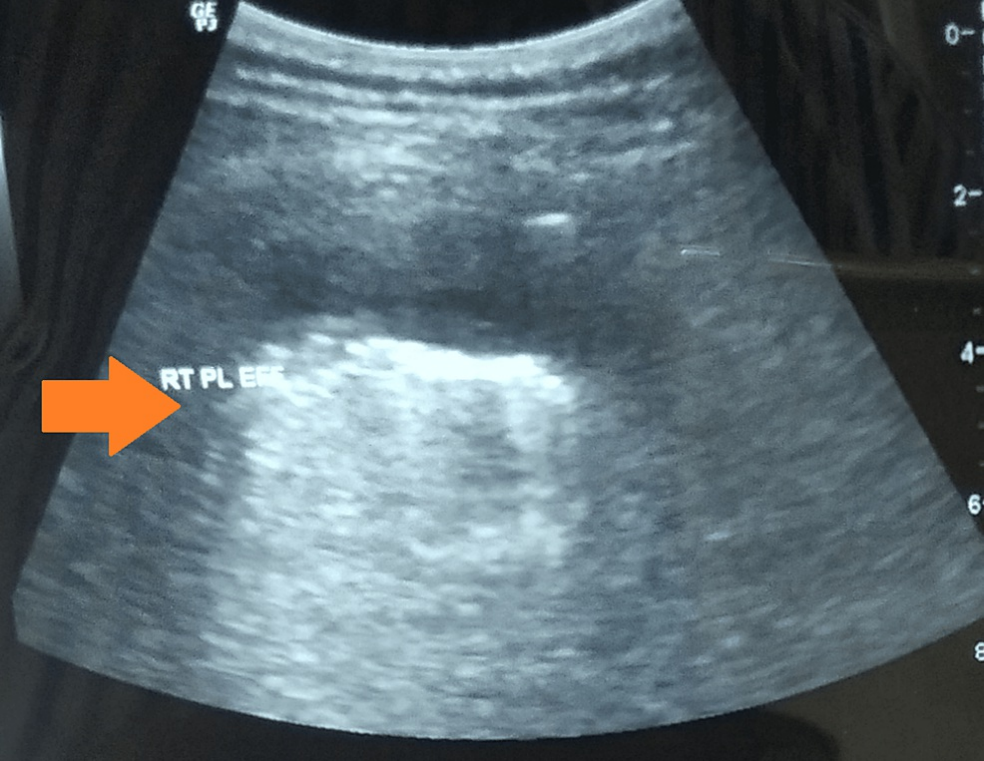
USG-chest revealing a 70.8 X 59.1 X 12.5 mm pleural effusion (arrow) USG: ultrasonogram
